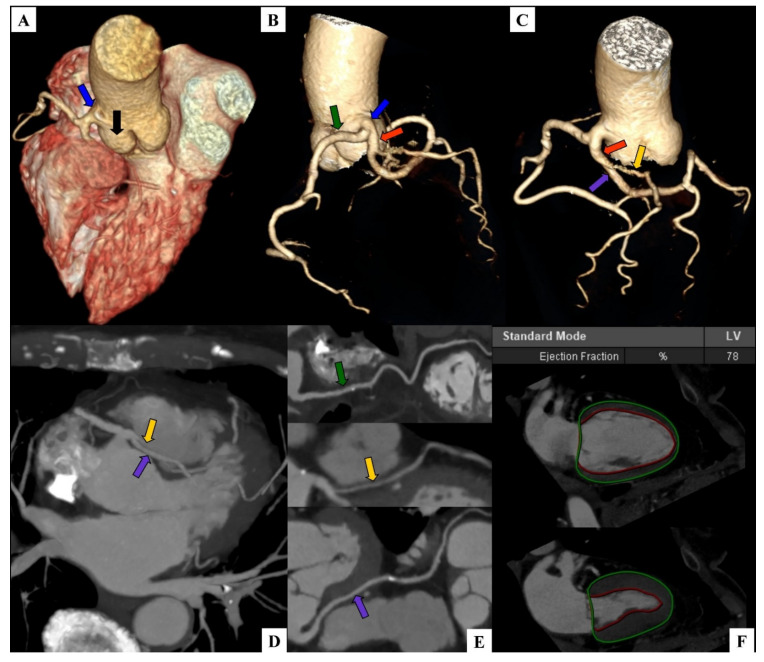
A single coronary artery originating from the right coronary sinus with a typical course of the right coronary artery and the interarterial course of the left main, left anterior descending, and left circumflex in coronary computed tomography angiography. On all panels, the arrows indicate: black—right coronary sinus, blue—single coronary artery, green—typical right coronary artery (RCA), orange—atypical left coronary artery (LCA), yellow—atypical left anterior descending artery (LAD), purple—atypical left circumflex artery (LCx). (A) Volume rendering technique (VRT). The origin of a single coronary artery from the right coronary sinus. (B) Volume rendering technique (VRT) with isolation of the vascular tree. Division of a single coronary artery into a typical RCA and an atypical LCA. (C) Volume rendering technique (VRT) with isolation of the vascular tree. Division of an atypical LCA into an atypical LAD and an atypical LCx. (D) Maximum intensity projection (MIP). Axial view. The interarterial course of LAD and LCx (E) curved planar reformation (CPR). Sequentially from the top: typical course of a right coronary artery, atypical course of a left anterior descending artery, atypical course of a left circumflex artery. (F) Left ventricular functional assessment. Left ventricular ejection fraction (EF)—78%.
